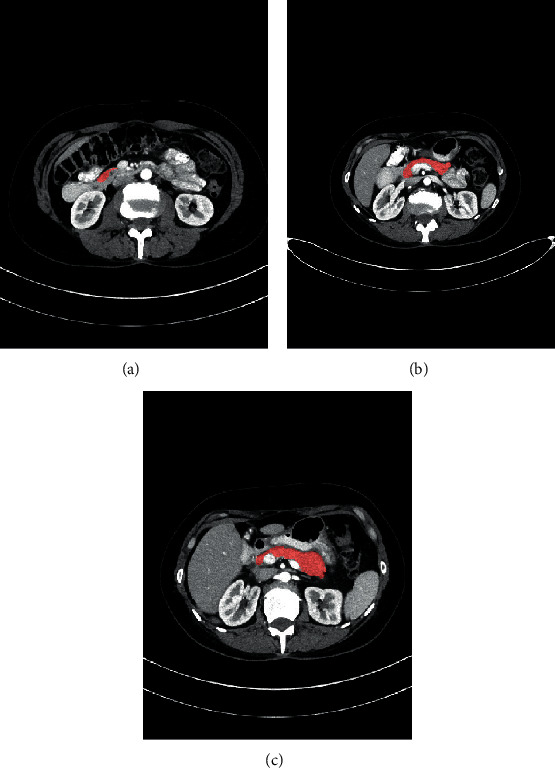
Examples of 2D CT slices with pancreas annotations (red regions), showing the highly variable shape and size of pancreas. The largest area of pancreas is less than 0.8% of entire slice while the smallest area is less than 0.1% (best viewed in color).
